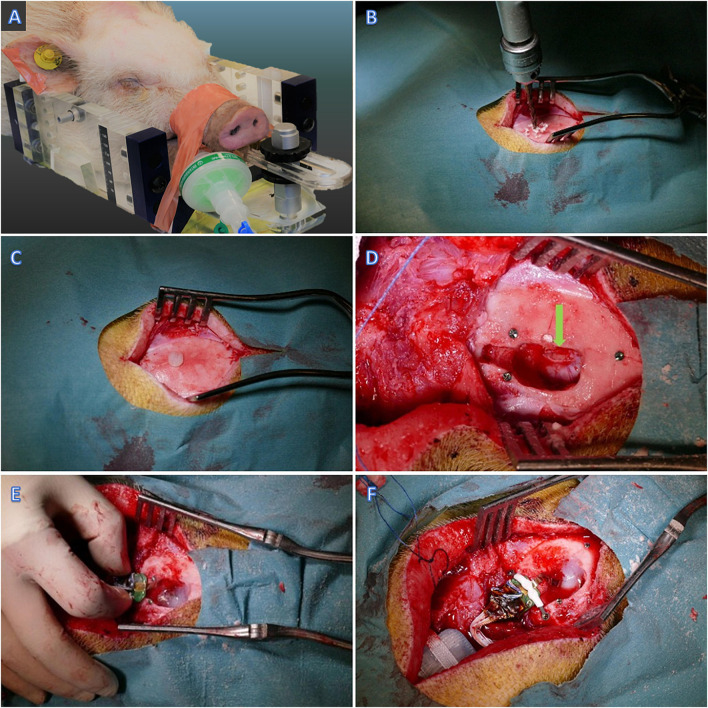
Implantation surgery. (A) The minipig fixated in the MRI compatible head frame. (B) Drilling the skull posterior to Bregma (C,D) Plastic and titanium fiducial markers. (D) Visual cortex exposure – pointed by green arrow. (E) Insertion of the probe in the visual cortex and fixation with Fibrin Sealant. (F) Fixation of the Omnetics connector to the skull bone.
